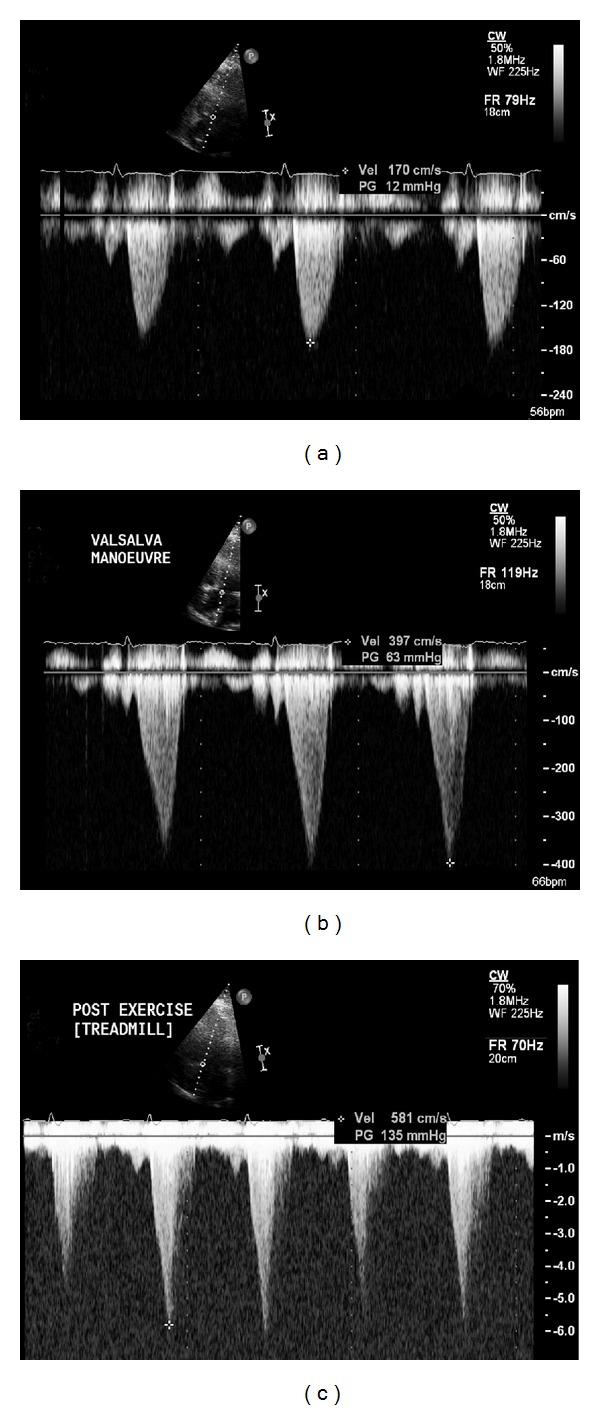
A 47-year-old man with hypertrophic cardiomyopathy underwent exercise SE. At rest, peak gradient across the aortic valve was 12 mm Hg (panel (a)). Repeat assessment during a held Valsalva manoeuvre revealed that the peak gradient was now 63 mm Hg (panel (b)), which indicates that exercise-induced LVOT obstruction is highly likely. This was indeed confirmed that at peak stress, the maximum gradient had increased to 135 mm Hg (panel (c)). Note the late systolic peaking of the Doppler profile in panels (b) and (c), indicative of dynamic rather than fixed outflow tract obstruction. The patient was commenced on beta-blocker therapy following the test results.
